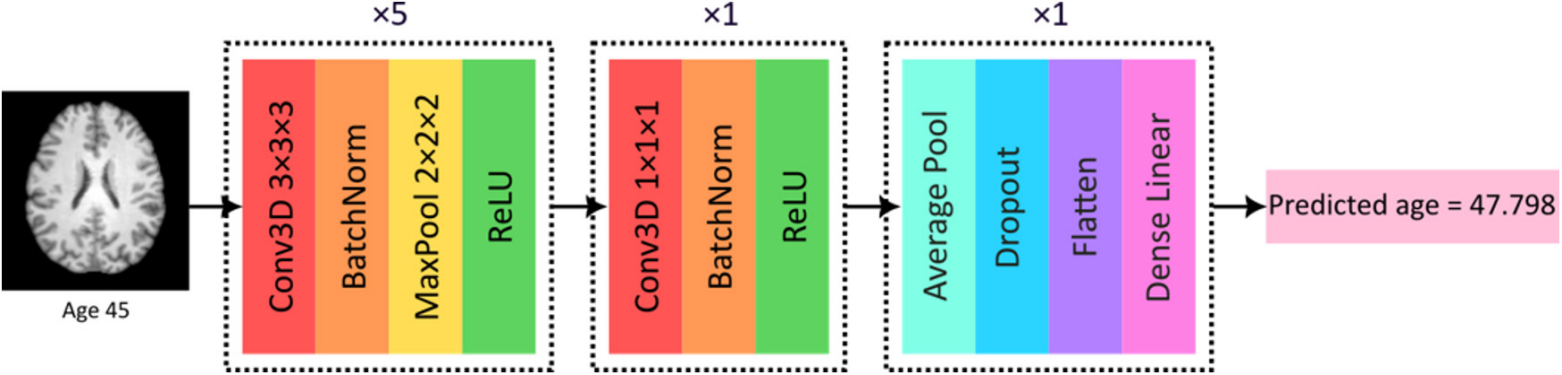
CNN model used in this work for biological brain age prediction using T1-weighted MRI. BatchNorm, batch normalization; Conv3D, 3D convolutional layer; MaxPool, max pooling; ReLU, rectified linear unit. Reproduced with permission from “CNN model used in this work for biological brain age prediction using T1w MRI” by Elizabeth Mcavoy, Emma A. M. Stanley, Anthony J. Winder, Matthias Wilms and Nils D. Forkert, licensed under CC BY-NC-ND.
